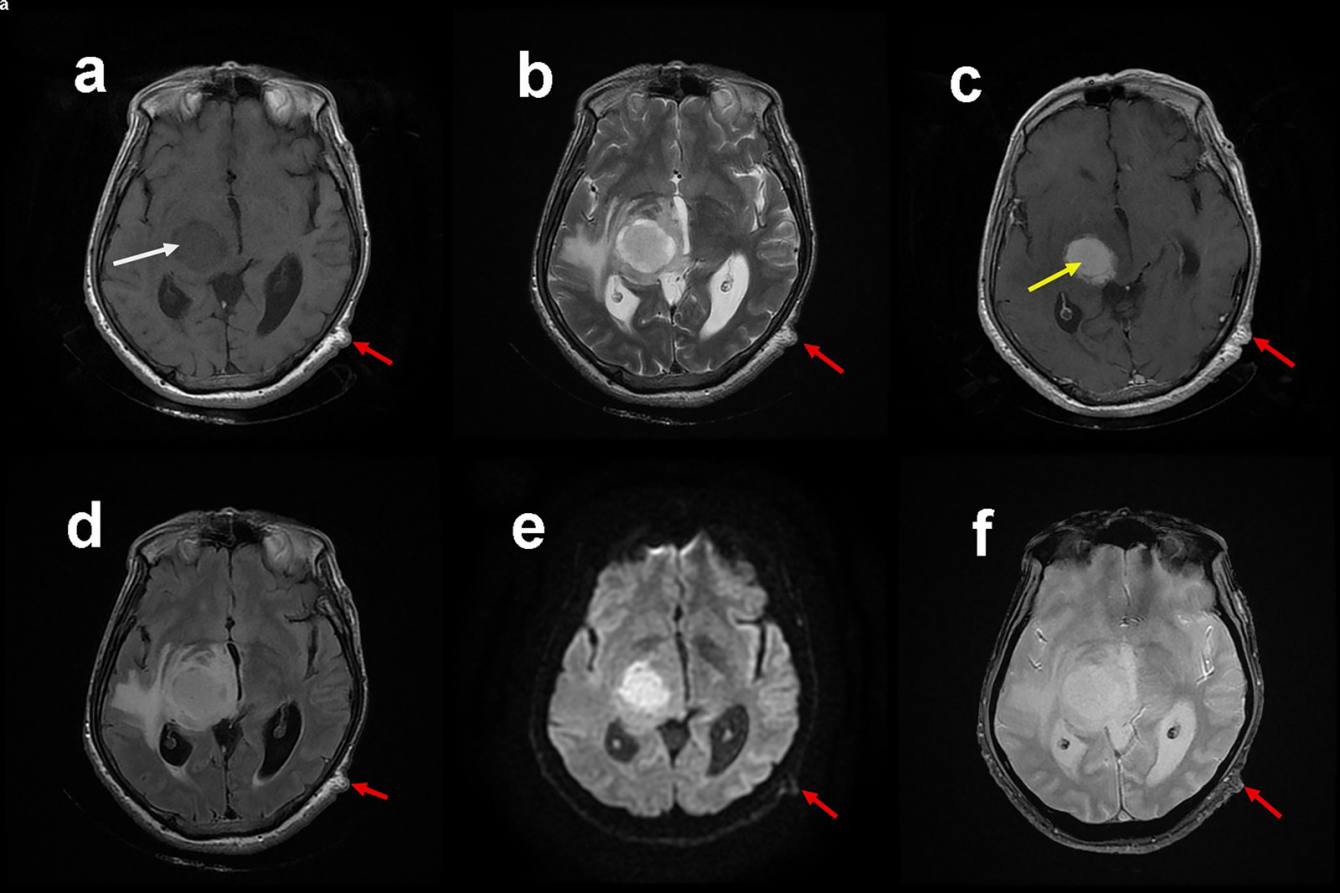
Axial MR images obtained at the time of clinical onset showing a right capsular and thalamic mass (white arrow), measuring 23 × 30 × 31 mm in long axes, hypointense on T1 weighted images (A) with heterogeneous hypersignal on T2 sequences (B), and which is intensely and homogeneously enhanced after injection of Gadolinium chelates having the aspect of a snowball (C; yellow arrow). Note the surrounded significant perilesional edema on T2 Fluid attenuated inversion recovery sequences (FLAIR) (D) exerting a mass effect on the ipsilateral ventricle, midline and the third ventricle. Diffusion-weighted imaging (DWI) revealed a moderate hypersignal (E). There was no evidence of bleeding on the gradient echo sequence (F). Note the neurofibroma of the scalp in the different sequences (red arrow). (Color version of figure is available online.)
